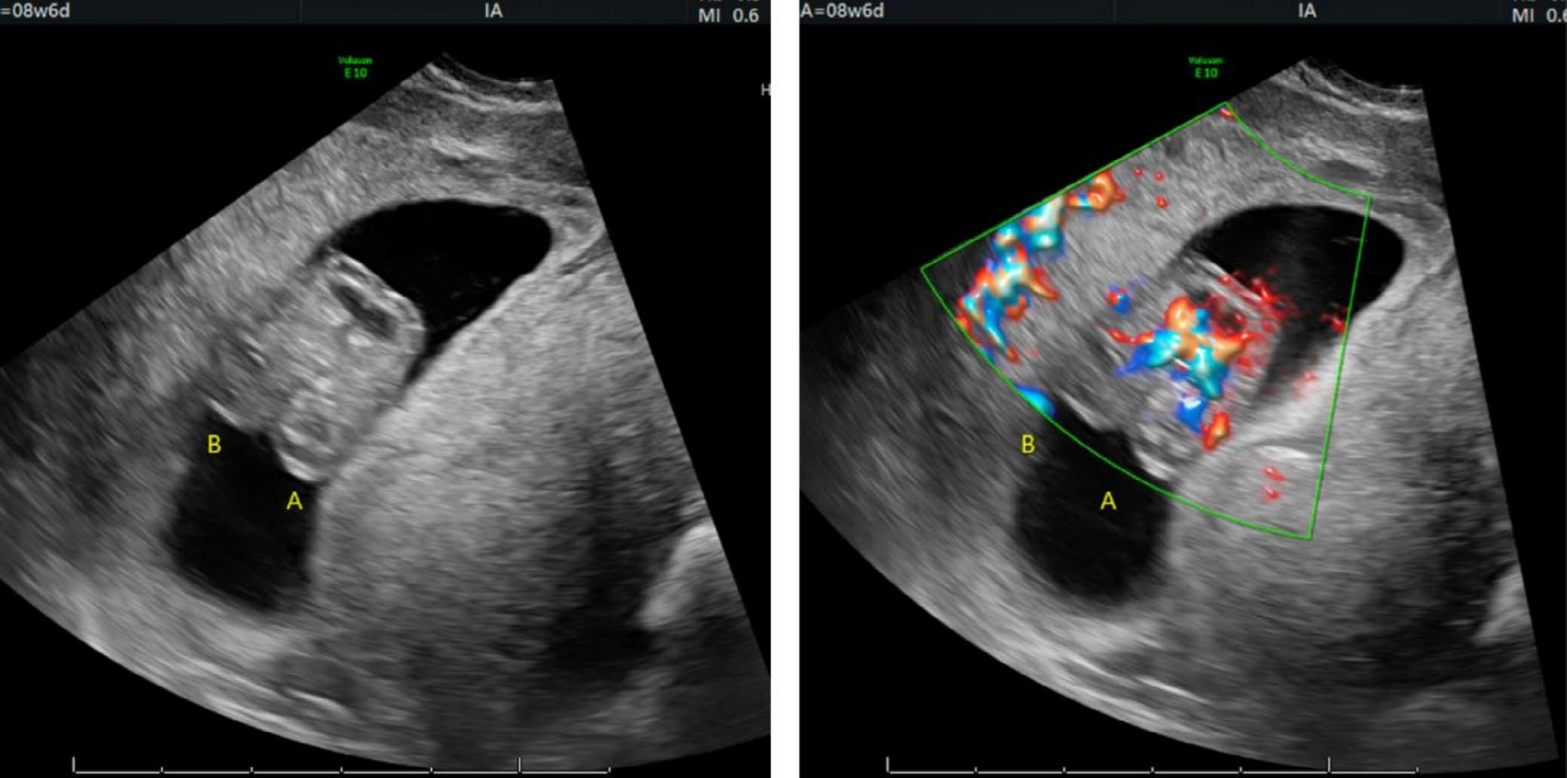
Thoraco‐omphalopagus conjoined twins at 8 weeks and 6 days with Doppler color flow demonstrating a shared heart.
